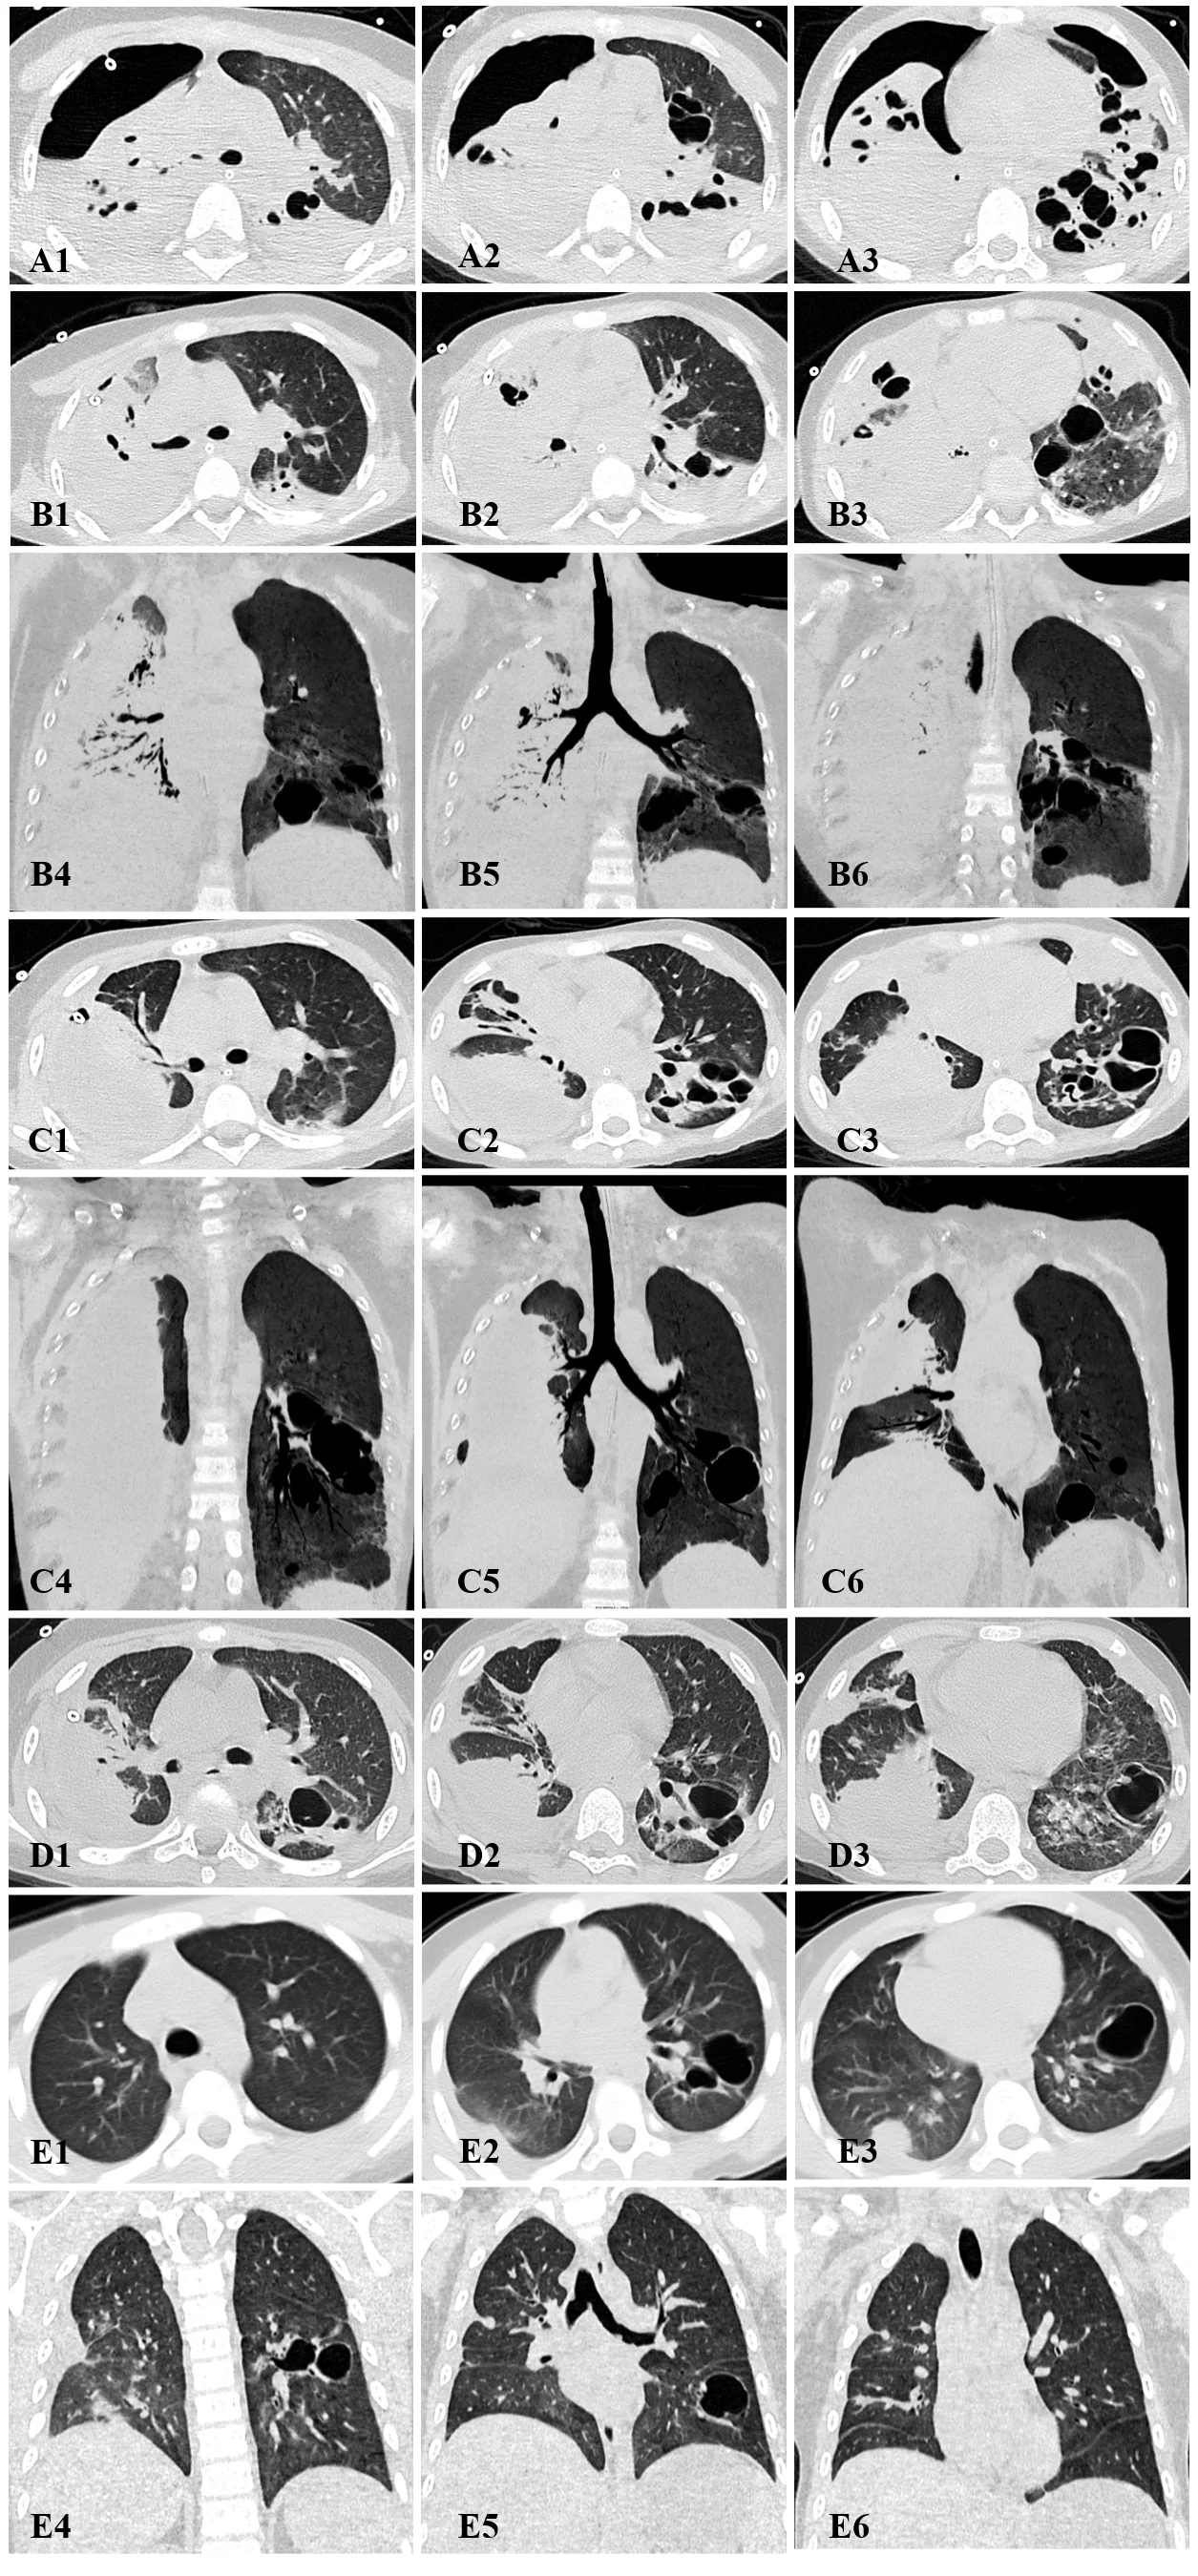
Chest computed tomography (CT) of the patient. (A1–A3) Bilateral pneumonia with multiple cavitary lesions and bilateral hydropneumothorax (the 17th day of admission). (B1–B6) The left-sided pneumonia had partially resolved, with a reduction in the number of cystic lesions in the left lower lobe, although some had increased in size; bilateral pleural effusions were present, and the bilateral pneumothorax had markedly diminished (the 30th day of admission). (C1–C6) The bilateral pneumonia had regressed, with a decrease in the number of cavities; right-sided hydropneumothorax was observed, and the left-sided pleural effusion had largely resolved (the 42nd day of admission). (D1–D3) The bilateral pneumonia showed partial resolution, with a reduction in the size of the multiple cavities (the 50th day of admission). (E1–E6) The bilateral pneumonia had significantly improved, with the multiple cavities in the left lung being reduced in both number and size (the 38th day after discharge).
